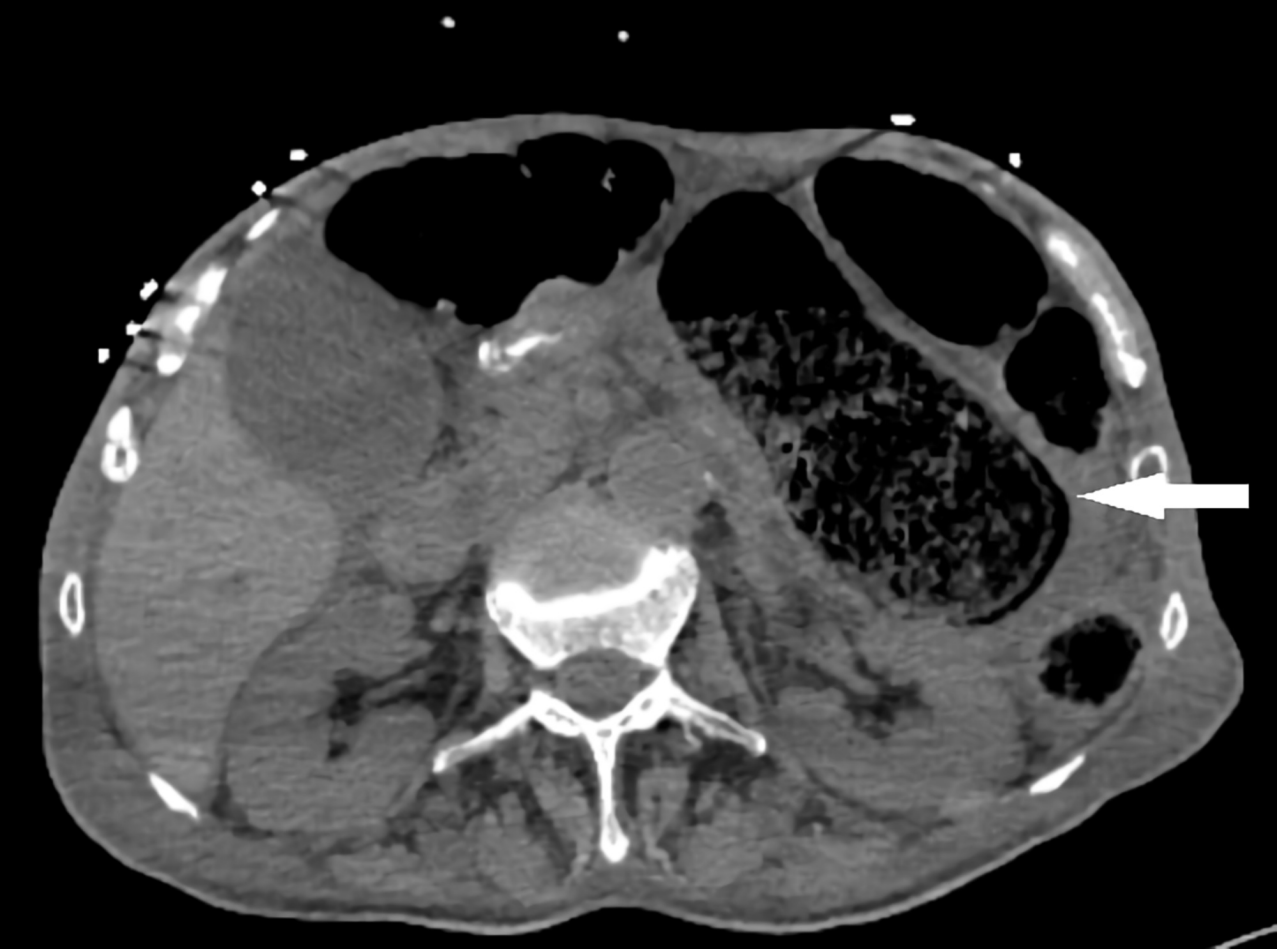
Dilated colon in computed tomography
